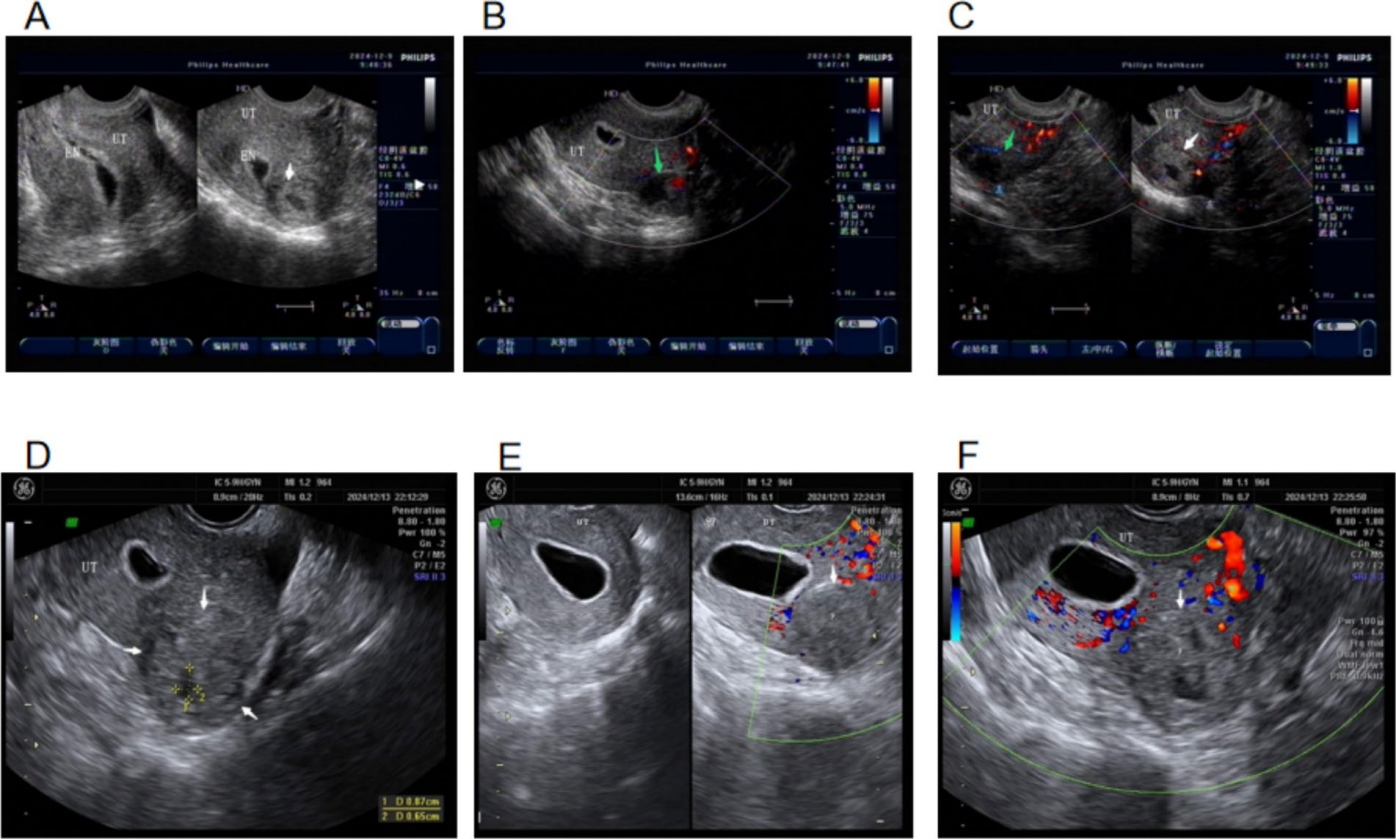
Ultrasound on days 22 and 26 of treatment. (A) White arrow: left interstitial tubal pregnancy. (B) Green arrow: partial thrombus dissolution within UAP. (C) UAP and left interstitial tubal pregnancy. (D, E) White arrows: mixed mass formed by miscarriage of the left interstitial tubal pregnancy; UAP disappeared. (F) White arrow: local magnified view of the mixed mass formed by miscarriage of the left interstitial tubal pregnancy.
